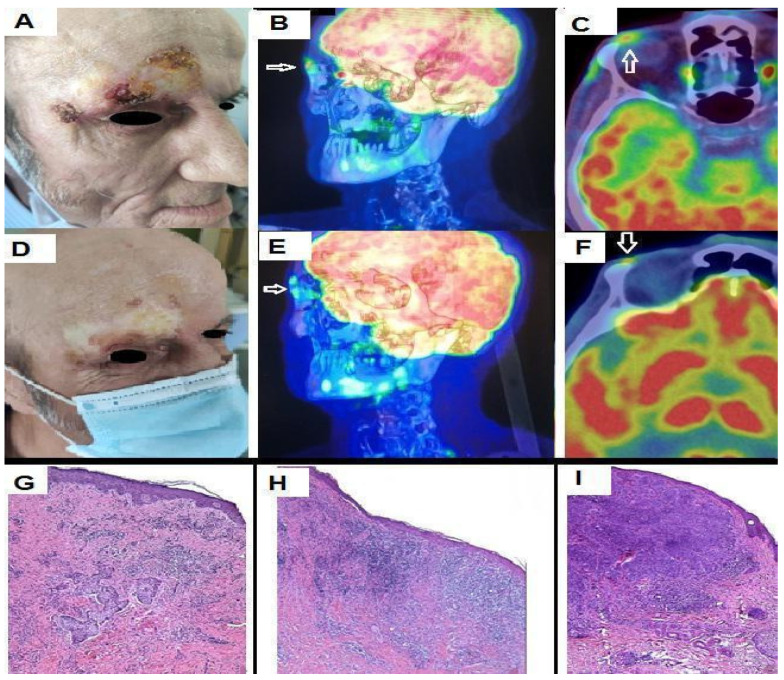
At the right fronto-orbital level, we observe an irregularly shaped, broad plaque with several shallow crusts (A). As indicated by arrows, PET/CT with 18F-FDG shows focal increased tracer uptake corresponding to the supraorbital lesion at presentation (B,C). After five therapeutic cycles, the patient exhibits significant clinical improvement (D). Also, PET/CT demonstrates a significant reduction in tracer incorporation (E,F). Section of an incisional biopsy from a large skin lesion showing an invasive cSCC before adjuvant treatment with Cemiplimab (G). A section of skin from the same area after medical treatment was negative for residual SCC and diffuse dermal inflammatory infiltrate (H). Residual BCC (I).
